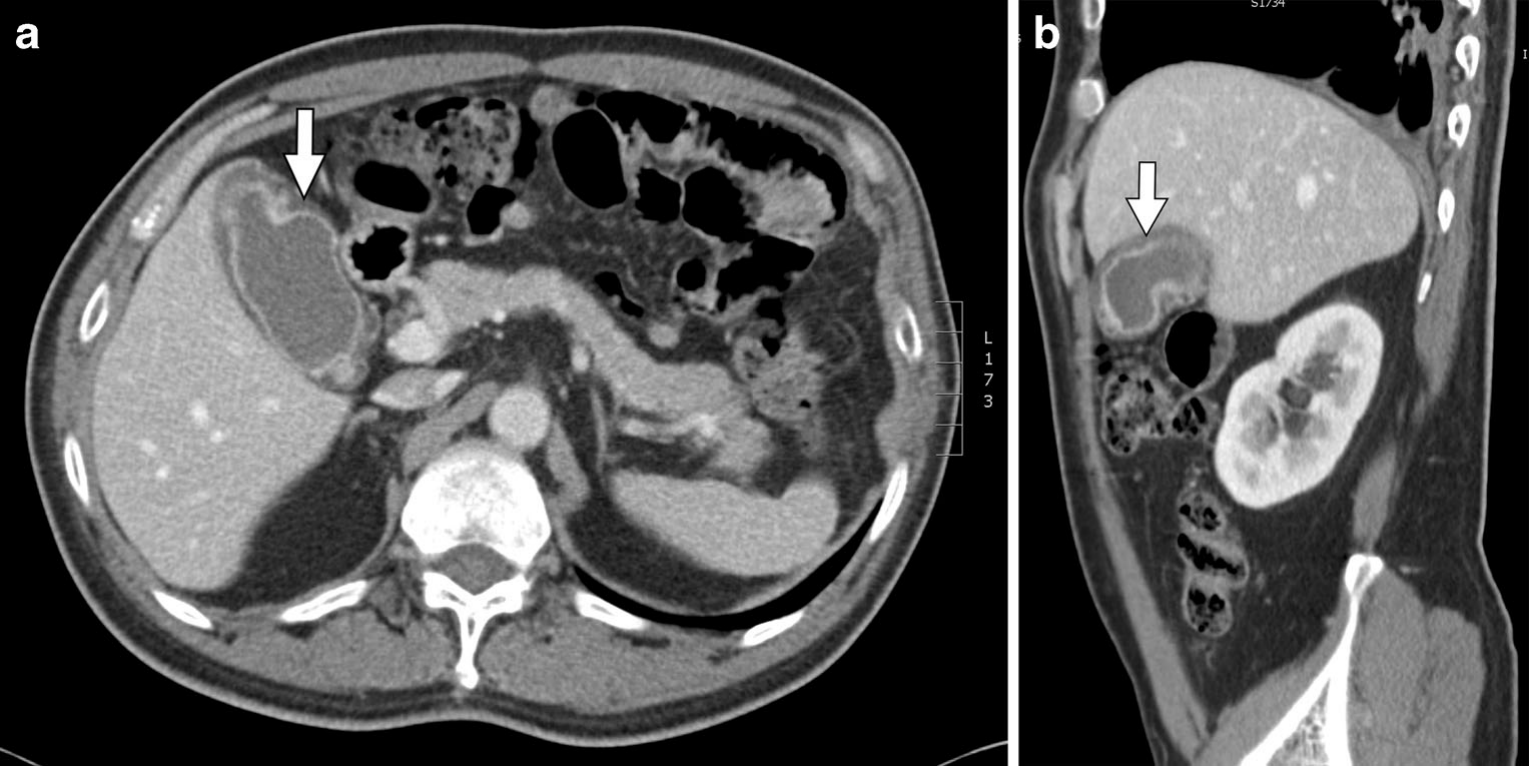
A 54-year-old male with stage IV non-small cell lung cancer status post chemotherapy treatment with cisplatin and premetrexed. a, b Axial and sagittal contrast-enhanced CT images, respectively, show a distended gallbladder with diffuse gallbladder wall thickening (*arrows*) consistent with acute acalculous cholecystitis
